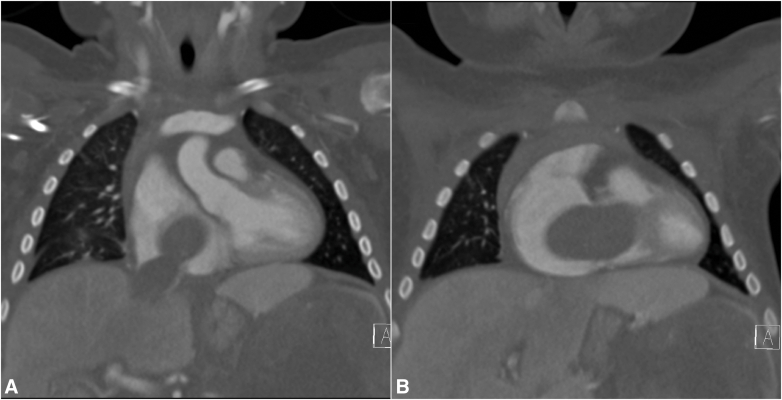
Preoperative computed tomography of patient 3. Coronal contrast-enhanced computed tomography images showing a large left renal mass with intravascular extension (A) reaching the right atrium and (B) right ventricle.
